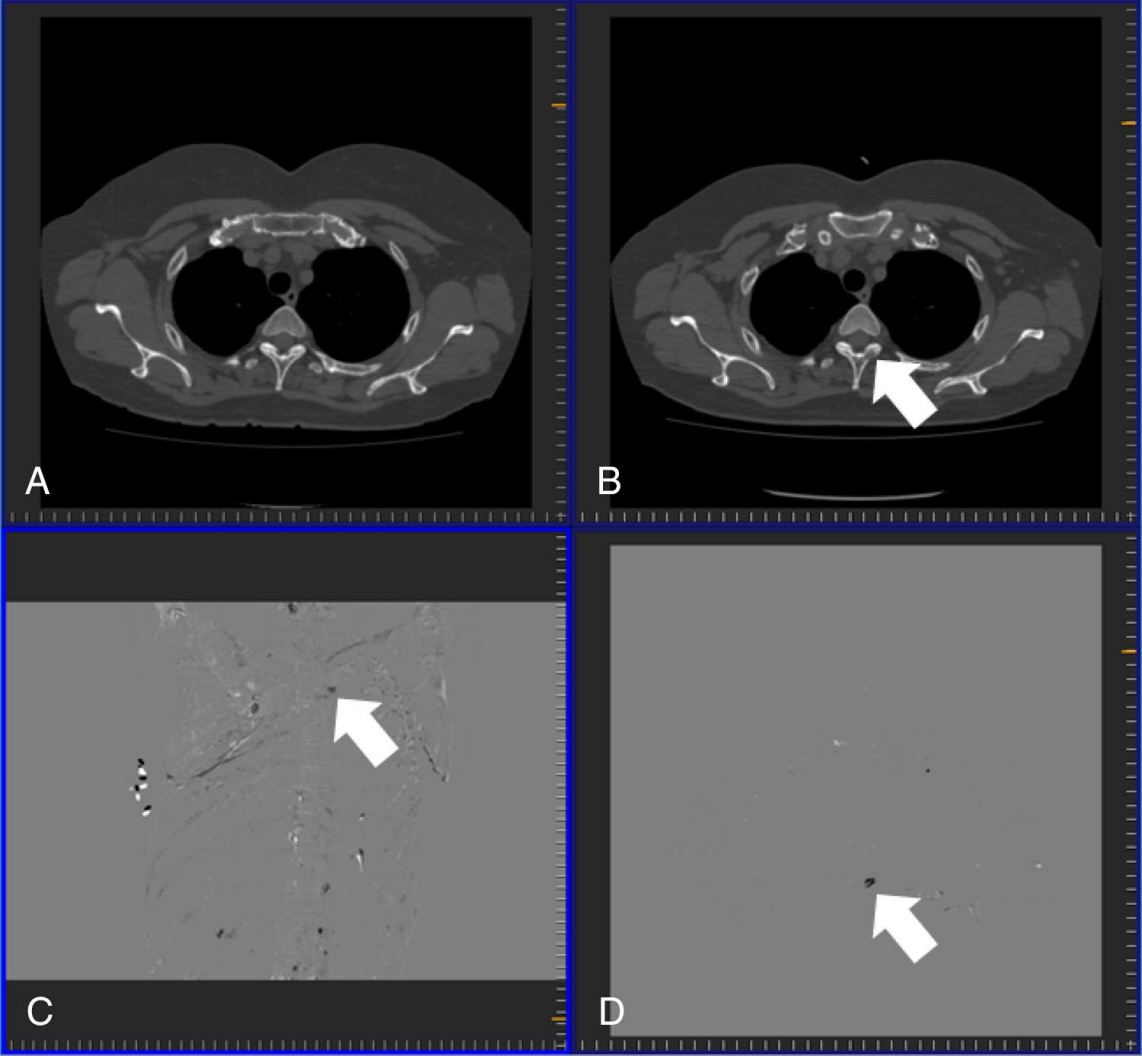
Osteolytic metastasis in the Th3 vertebra (arrow) of a 54-year-old female patient with breast cancer. (A) Previous computed tomography (CT) image; (B) current CT image; (C) temporal subtraction (TS) image; (D) projection image of TS images.
